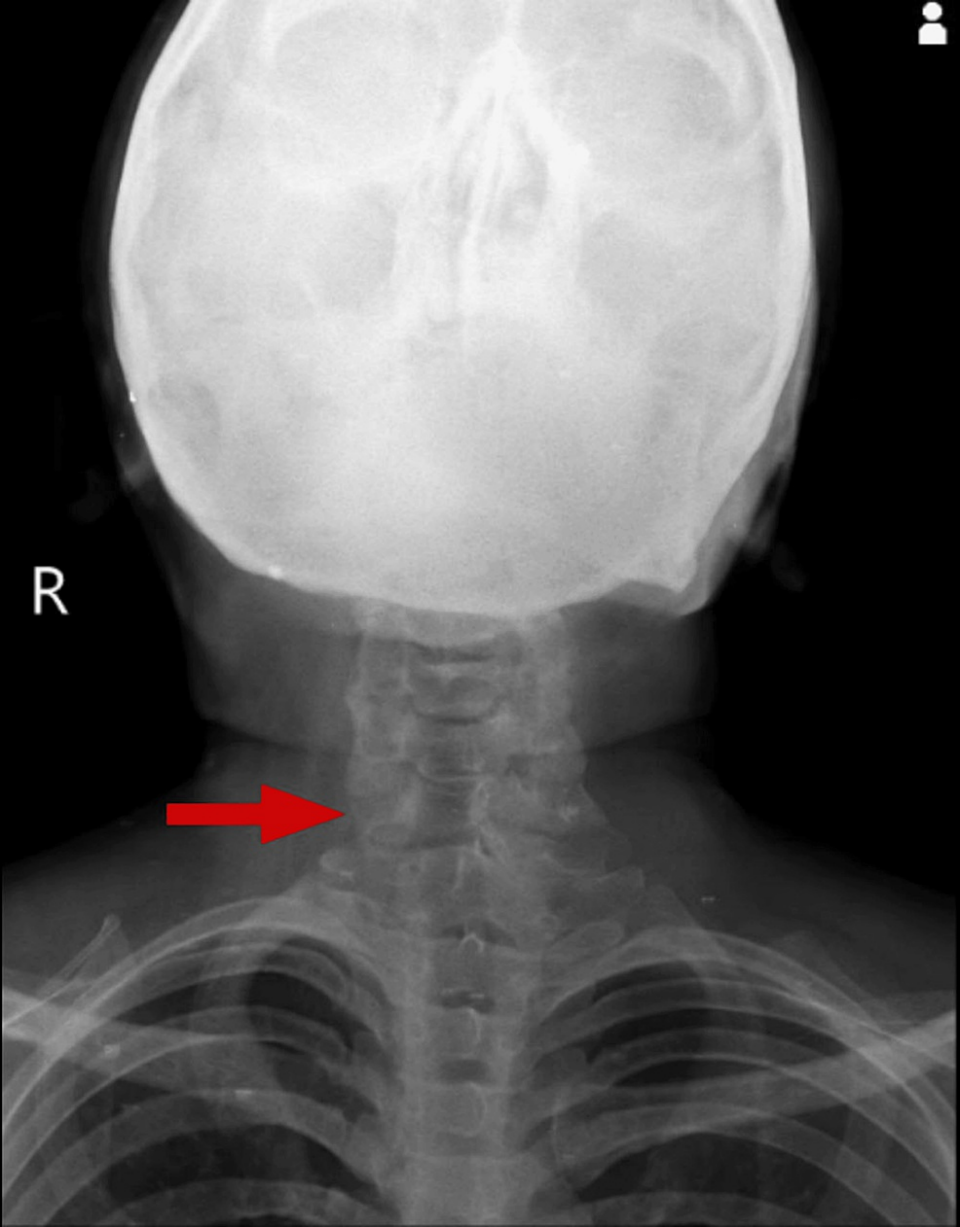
Posterior X-ray of the cervical spine with reduced space between C1 and C7 vertebrae
